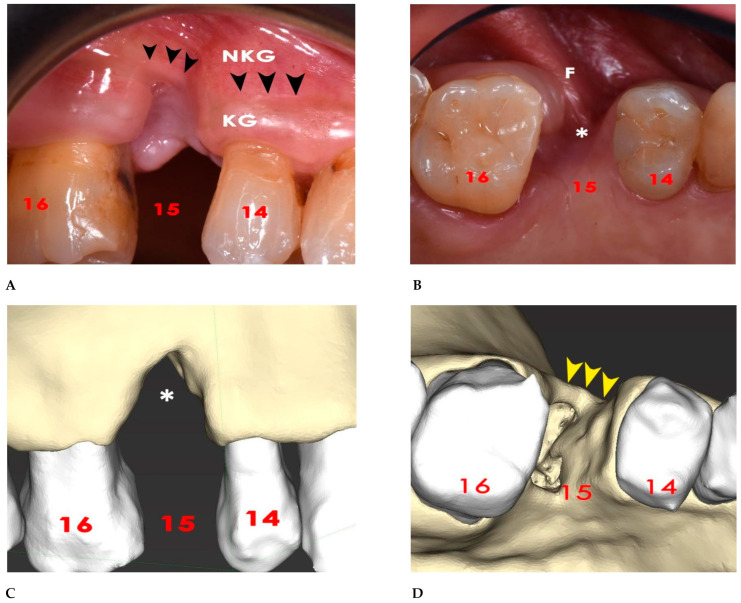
(A) At the site of tooth 15, keratinized gingiva (KG) was lost up to the junction with the non-keratinized gingiva (NKG). Arrows indicate the junction between the KG and NKG. (B) A frenulum (F) was inserted in the apical part of the gingival defect (*). (C) The 3D bone defect model resulting from the DICOM data of the baseline CBCT has a frontal “V”-shaped vertical defect with loosening of the entire alveolar process (*). (D) An occlusal view showed the horizontal extent of the bone alveolar defect (arrows).
